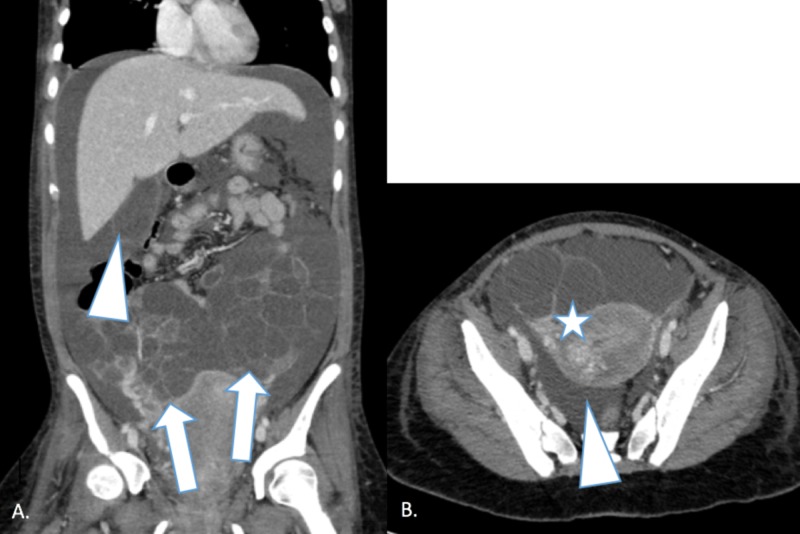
Computed tomography of the thorax, abdomen, and pelvis in a 19-year-old female showing findings of severe (grade 4) ovarian hyperstimulation syndrome. Image A - coronal computed tomography image showing multiple large bilateral cystic structures in the pelvis (arrows) with intra-abdominal and pelvic free fluid (arrowhead). Image B – axial computed tomography image showing heterogeneous enhancing material in the uterus (contained products of pregnancy) (inferior to star). These findings in images A and B are consistent with a nonviable partial molar intrauterine pregnancy and severe (grade 4) ovarian hyperstimulation syndrome.
